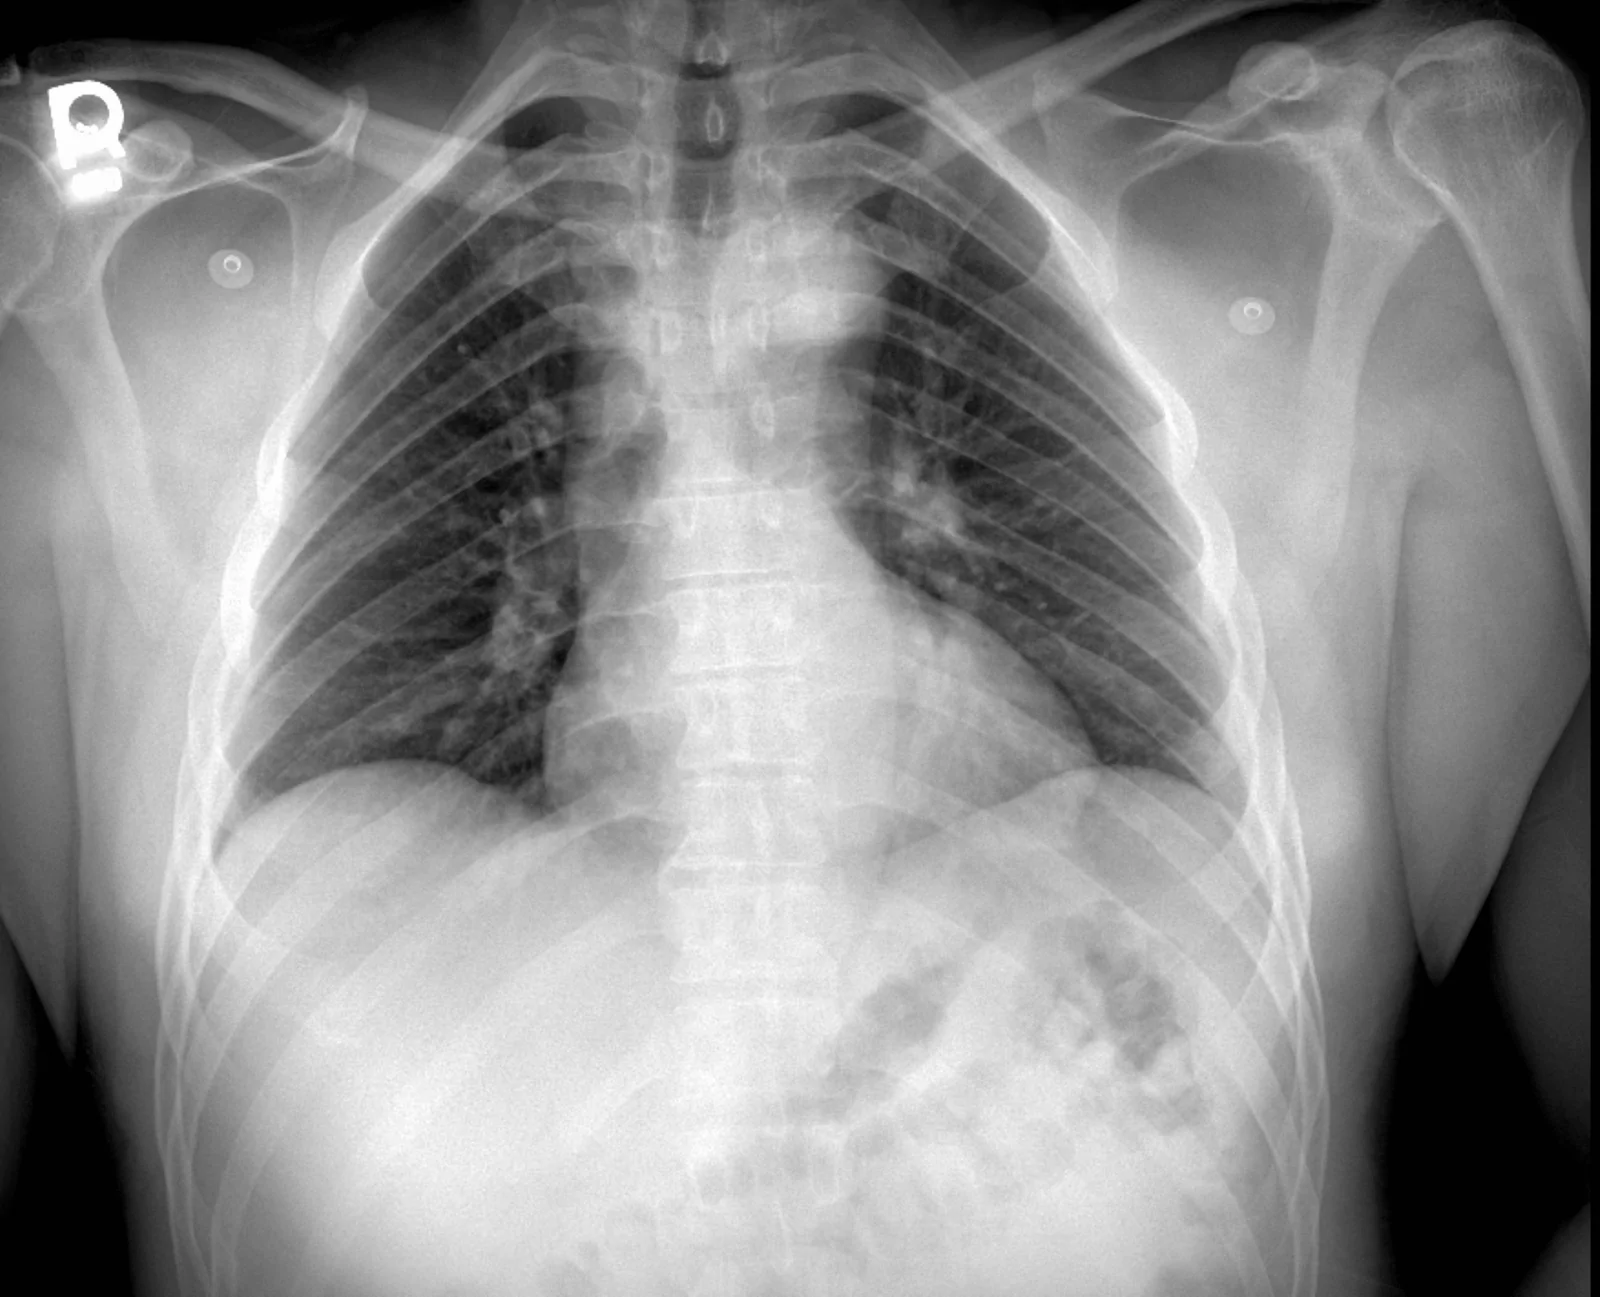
Chest Radiograph Demonstrating No Acute Cardiopulmonary Abnormalities
